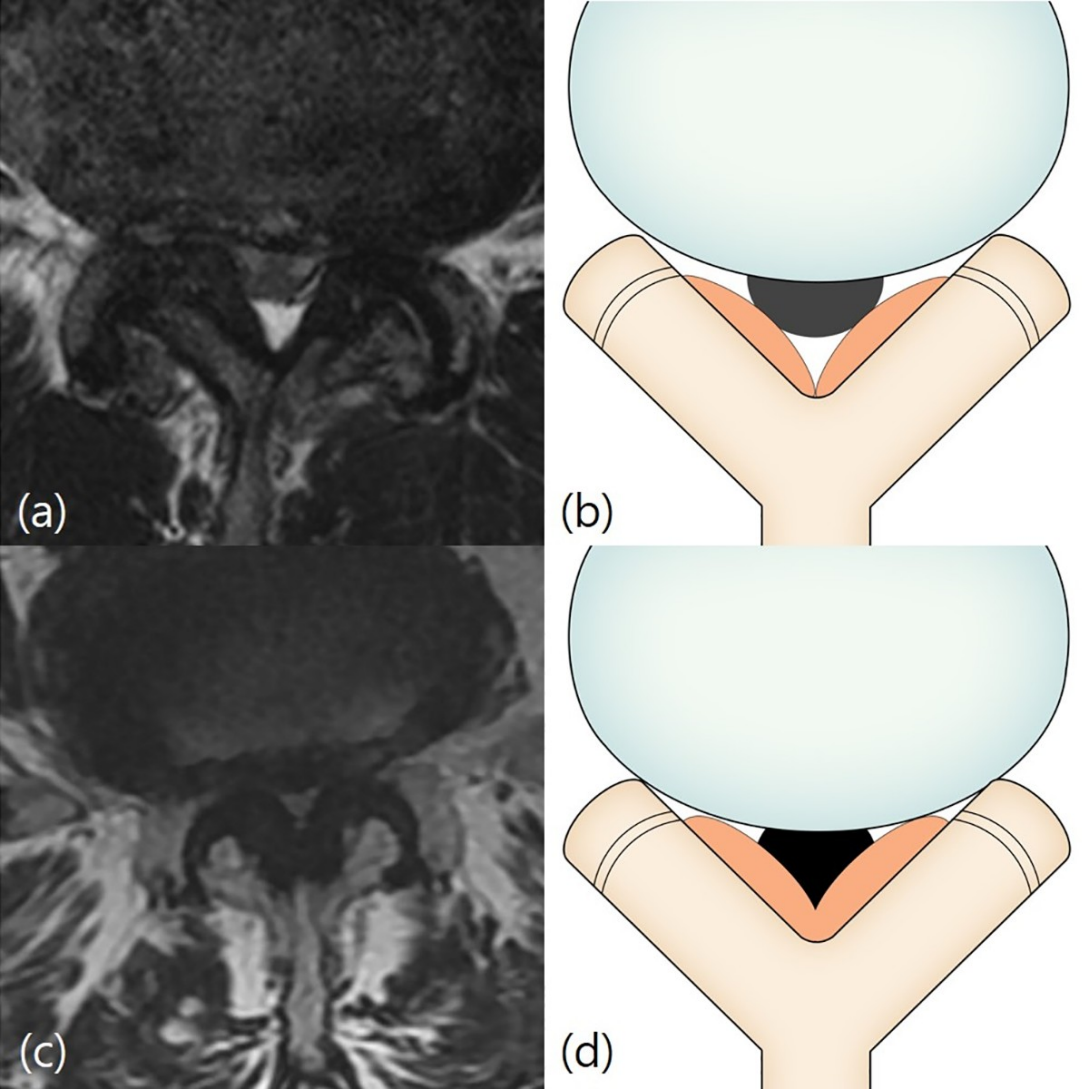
Schizas classification. Grade C and D (severe or extreme stenosis). Fig 3A and 3B: Grade C (severe stenosis). A 71-year-old man with left lower extremity radiculopathy. T2-weighted axial magnetic resonance image at L4/5 disc level shows that no recognizable rootlets with complete effacement of cerebrospinal fluid space, but epidural fat was present posteriorly. Fig 3C and 3D: Grade D (extreme stenosis). A 70-year-old woman with right lower extremity radiculopathy. T2-weighted axial magnetic resonance image at L4/5 disc level shows no recognizable rootlets and no epidural fat posteriorly.
